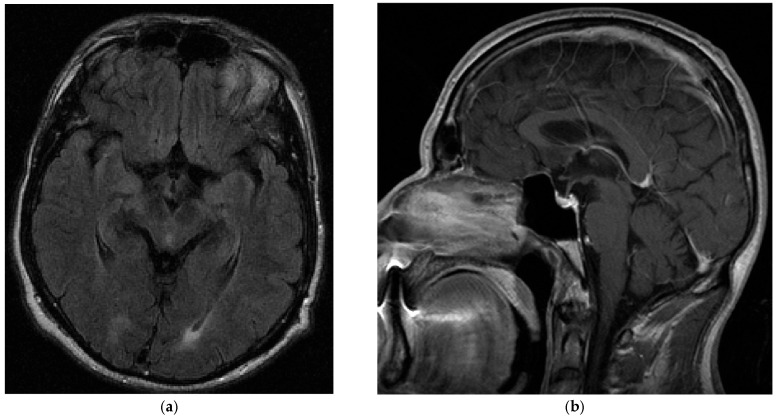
Cerebral MRI at baseline. (a) Axial fluid attenuated inversion recovery (FLAIR) sequences showing normal midbrain anatomy. (b) Sagittal contrast-enhanced T1-weighted sequences demonstrating normal brain stem anatomy.
